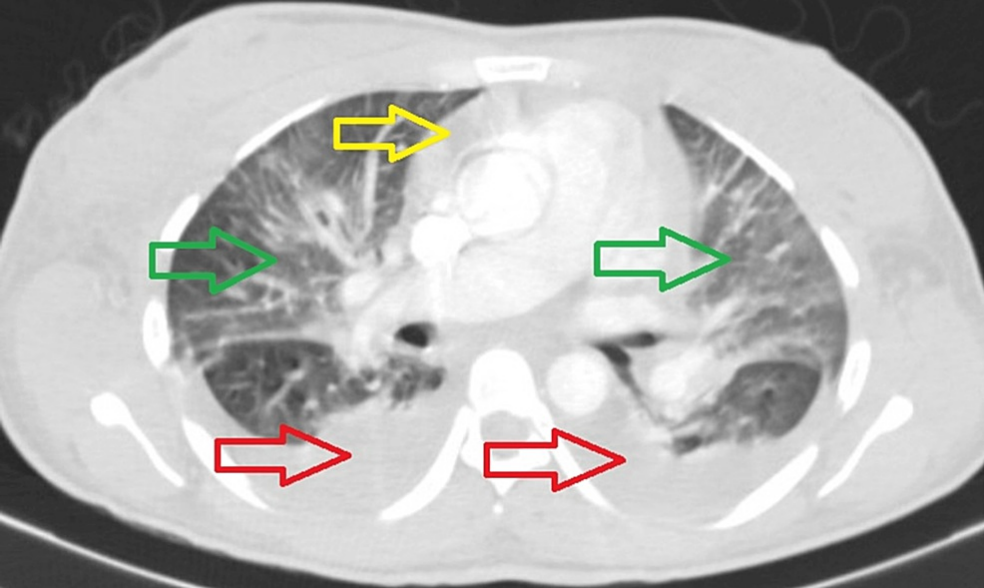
Chest CT scan showing bilateral pleural effusion (red arrows), ground-glass opacities (green arrows), and pericardial effusion (yellow arrow).
